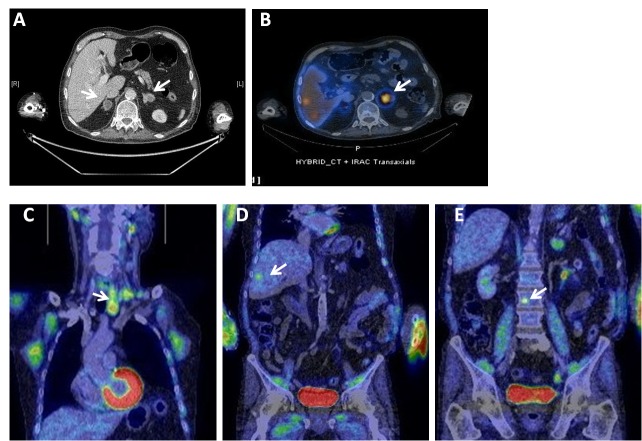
Computed tomography showed bilateral nodules (A) with the left adrenal gland showing avid uptake on the iobenguane scan in addition to liver deposits (B). Positron emission tomography showed uptake in the left lobe of the thyroid gland and cervical lymph nodes (C), segment V/VI of the liver (D) and a small deposit in the body of L3 (E).
